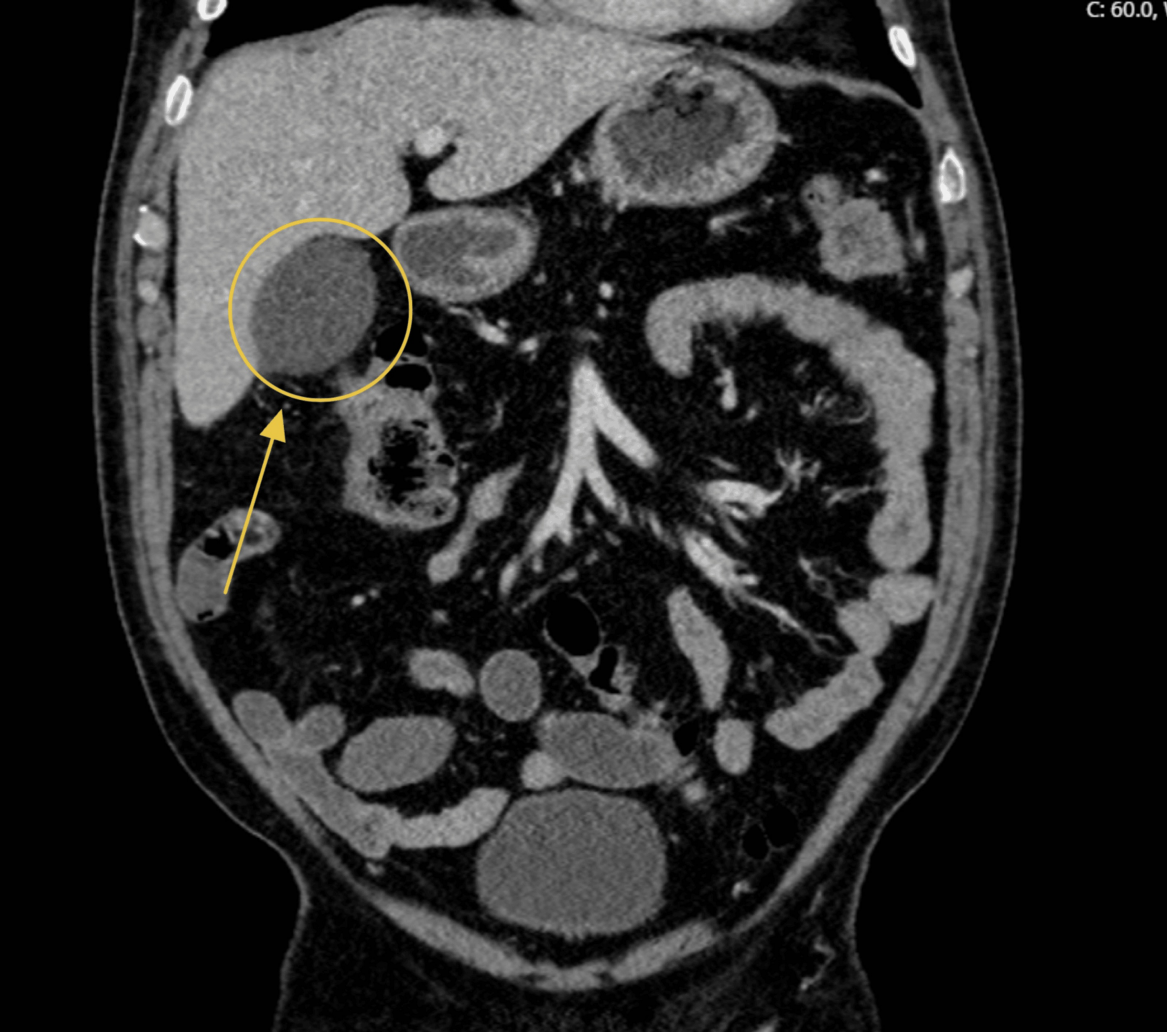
Distended gallbladder on CT
